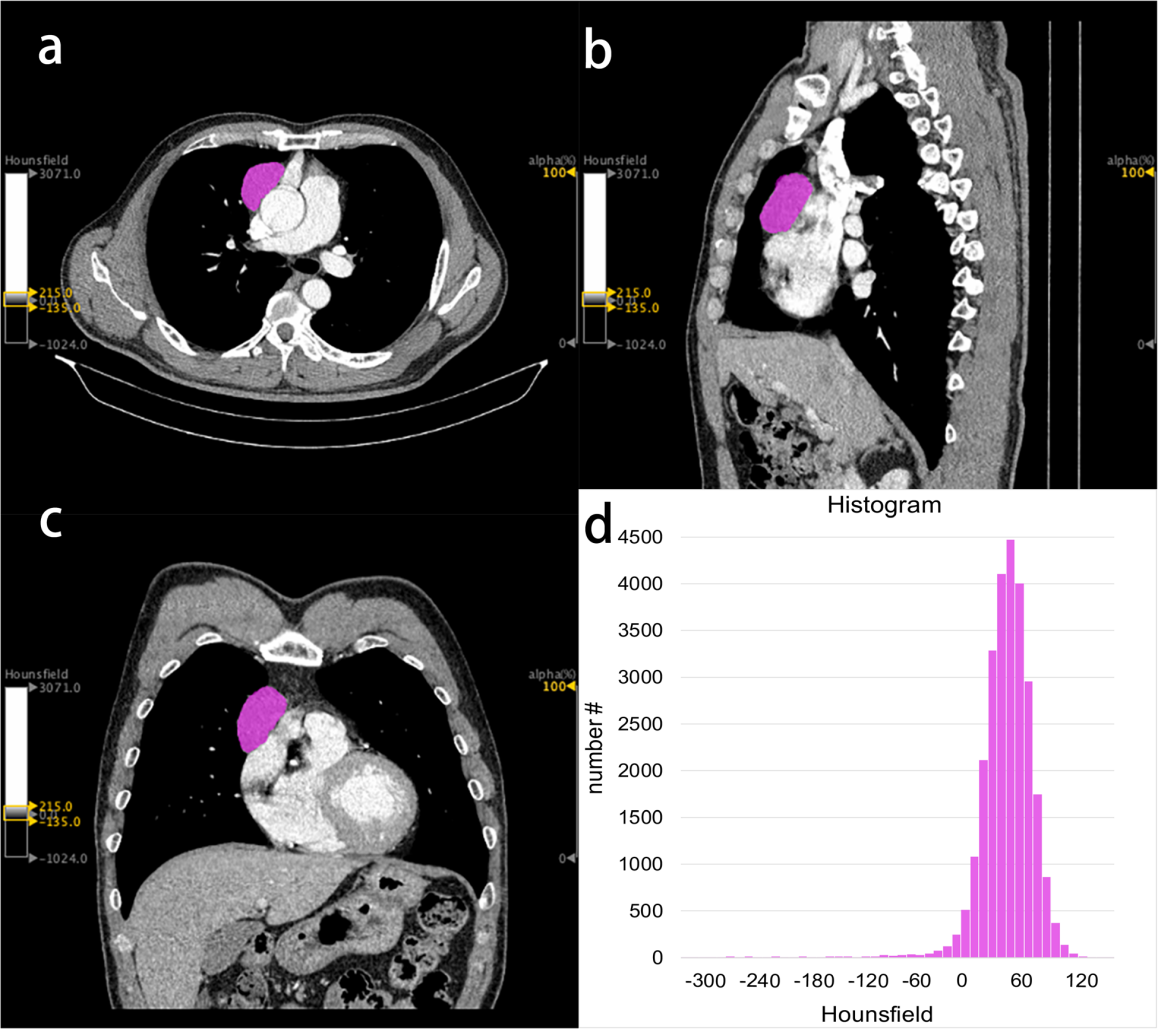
Chest enhanced CT images of a 53-years-old man with type B1 thymic epithelial tumor (low-risk TET). Texture features are extracted from the primary tumor area (purple overlay). a transverse section; b median sagittal section; c coronal section; d histogram
